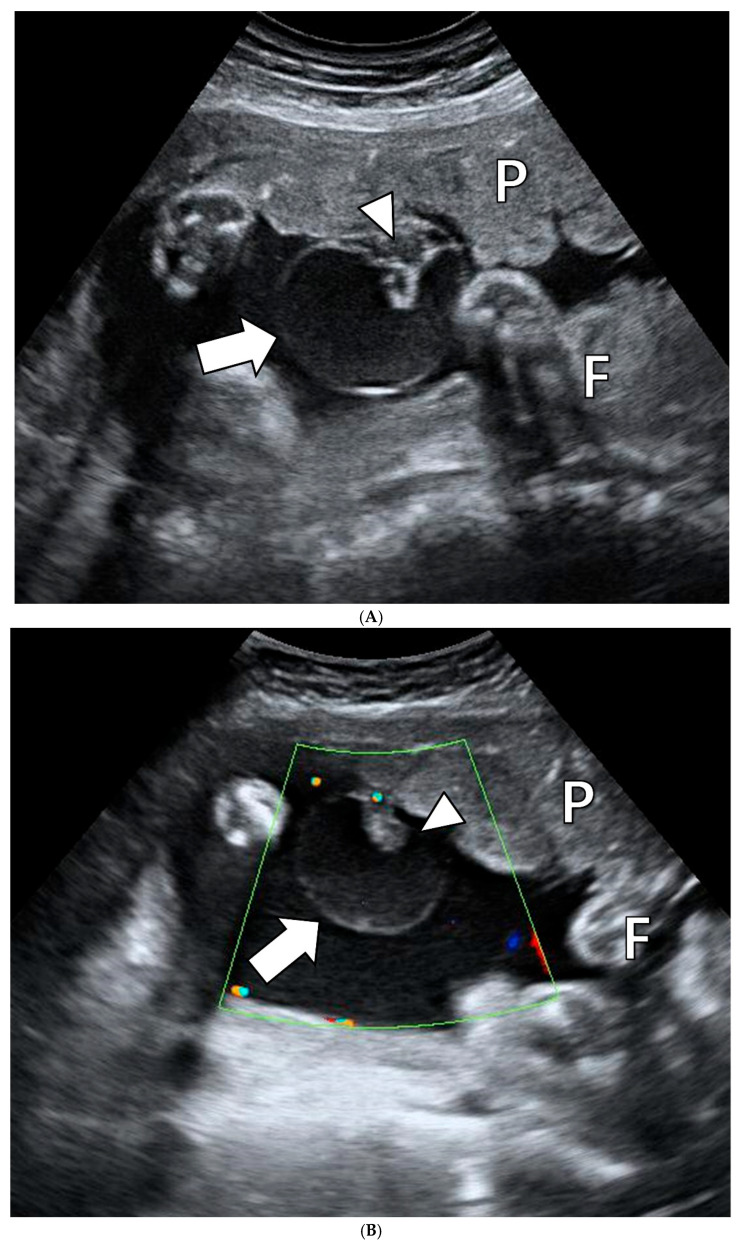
Prenatal ultrasonography of the subchorionic placental cyst at 35 weeks and 3 days of gestation. (A), An echolucent cystic mass is protruding from the fetal surface of the placenta (thick arrow). An intracystic heterogeneous echogenic lesion is shown (arrowhead). (B), Color Doppler images reveal no blood flow in the cyst. The cyst (thick arrow) and intracystic heterogeneous echogenic lesion (arrowhead). P, placenta; F, fetus.
